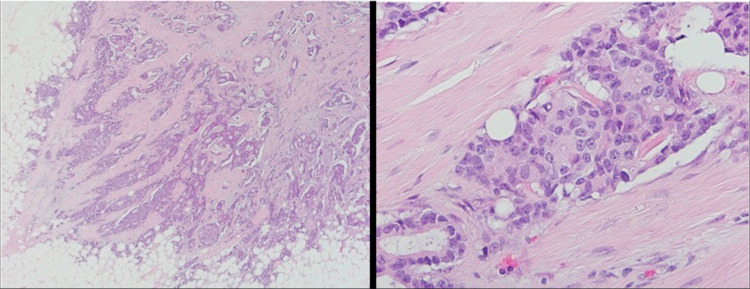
Ultrasound-guided biopsy of the left breast. Left image: low magnification showing invasive/neoplastic ductal cells, with surrounding fibrosis, going into adjacent fat. Right image: high magnification of neoplastic cells with nuclear changes, such as membrane irregularity and prominent nucleoli.
